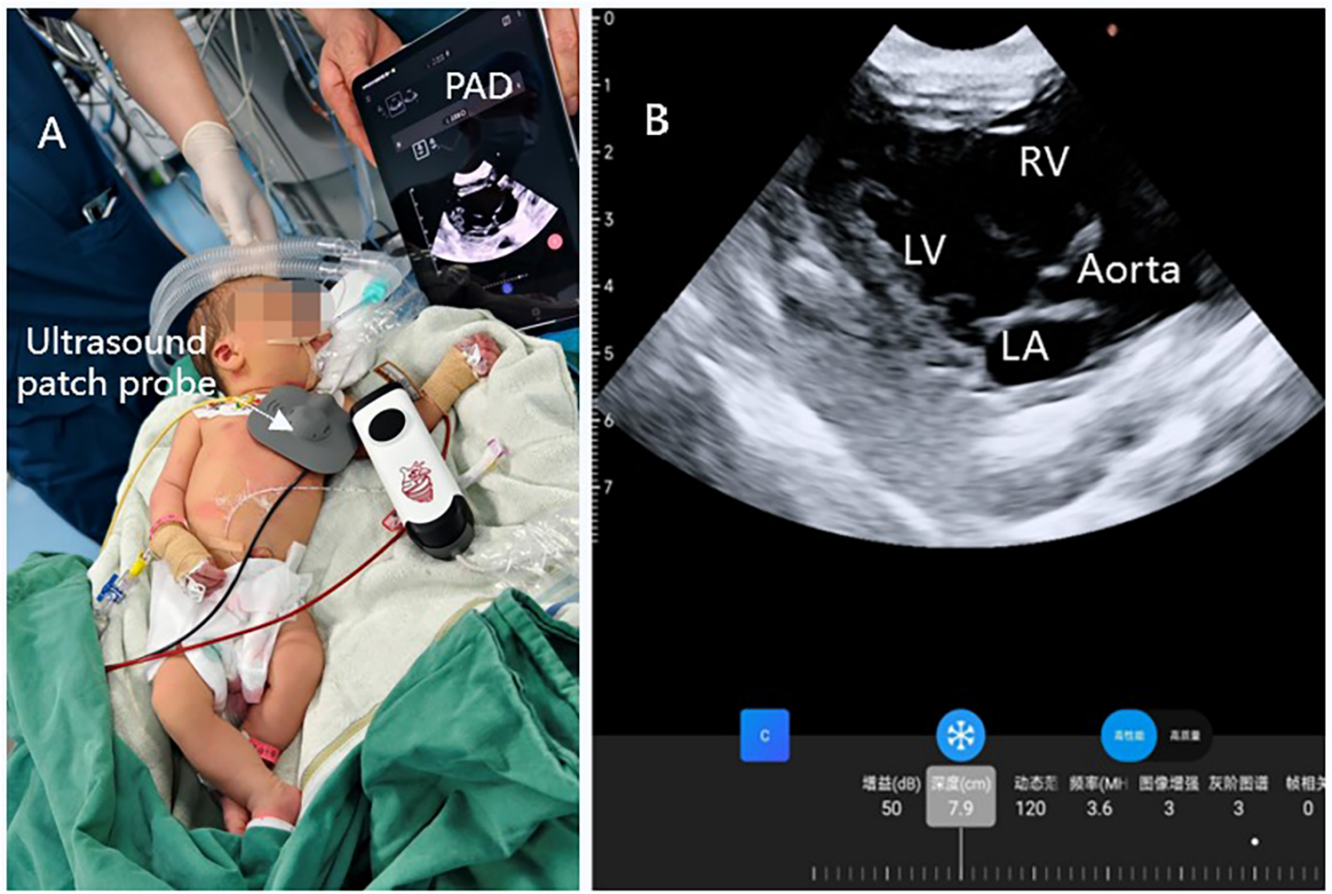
Continuous ultrasound imaging of the heart during operation. (A) Wearable ultrasound technology is utilized in anesthesia within clinical settings; (B) continuous ultrasound imaging of parasternal long-axis view.
